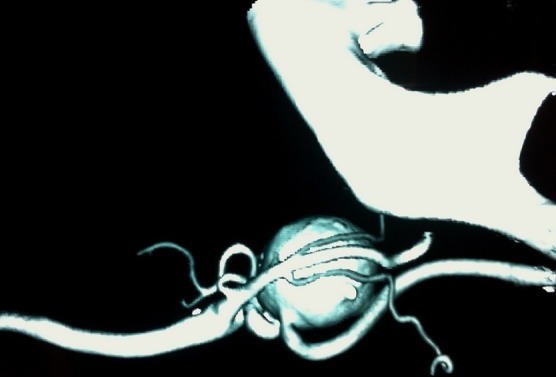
Reconstructed angio-CT scan showing a saccular aneurysm originating from the proximal left ICA
